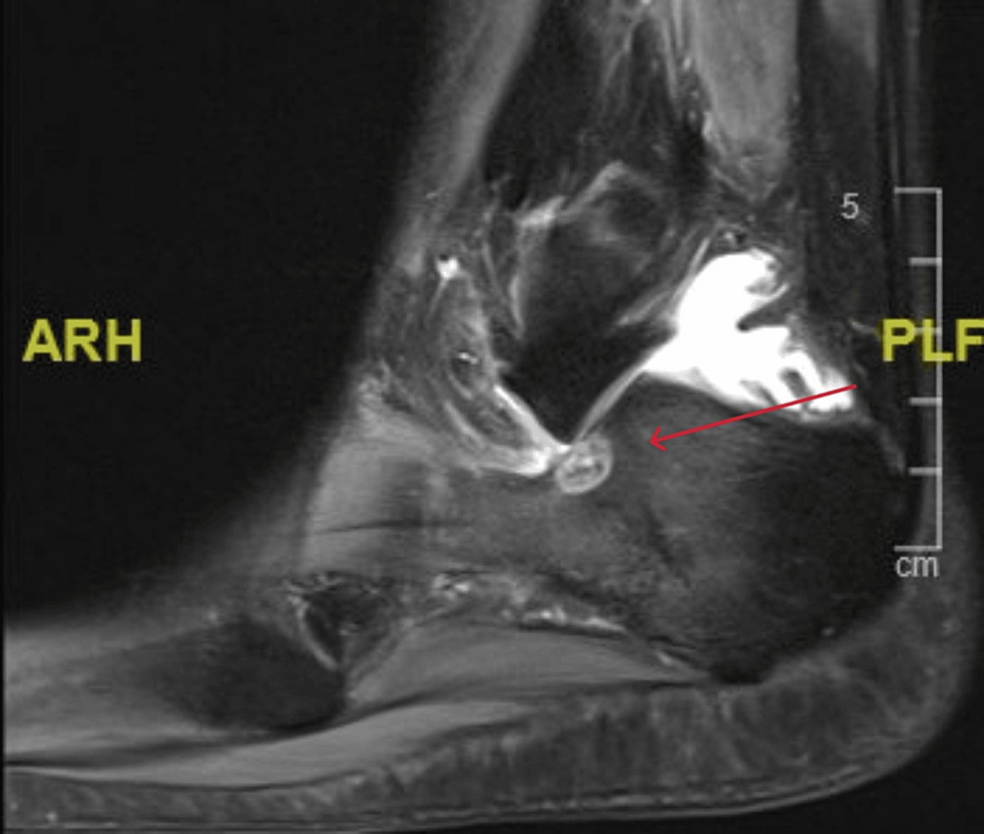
Sagittal view MRI T2 window of the right ankle at the time of presentation MRI: magnetic resonance imaging
